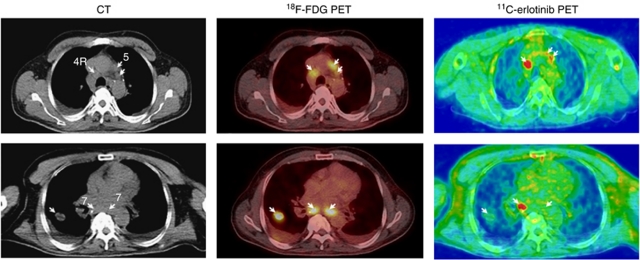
^11^C-erlotinib PET/CT demonstrates the heterogeneous nature of advanced lung cancer. Two transaxial slices of (left) contrast-enhanced CT; (middle) ^18^F-FDG PET/low-dose CT, and (right) ^11^C-erlotinib PET/low-dose CT. A 48-year-old patient (no. 7) with NSCLC in the right lung and enlarged mediastinal lymph nodes (upper and lower panel left figure, arrows). Both ^18^F-FDG and ^11^C-erlotinib accumulated in lymph nodes at positions 4R and 5 (upper panel, arrows). The tumour in the right lung and one of the lymph nodes at position 7 showed only a weak accumulation of ^11^C-erlotinib (arrows). The ratio between the ^11^C-erlotinib average radioactivity concentrations in the lymph node metastasis and that in surrounding lung tissue was 2 (see Figure 4).
